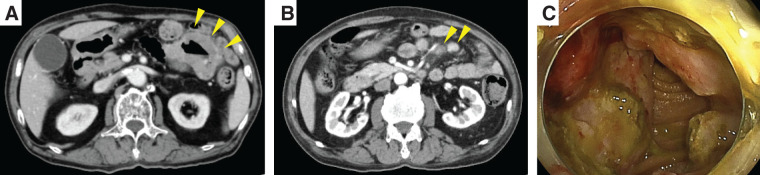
Images of contrast-enhanced CT and DBE. (A) CT images showing a circumferential, contrast-enhancing jejunal lesion near the duodenojejunal flexure (yellow arrowheads). (B) Multiple enlarged mesenteric lymph nodes along the tumor-feeding vessels (yellow arrowheads). (C) DBE demonstrating a 40-mm, circumferential, near-obstructive jejunal tumor just distal to the duodenojejunal flexure. Distal scope passage was impeded. DBE, double-balloon enteroscopy
